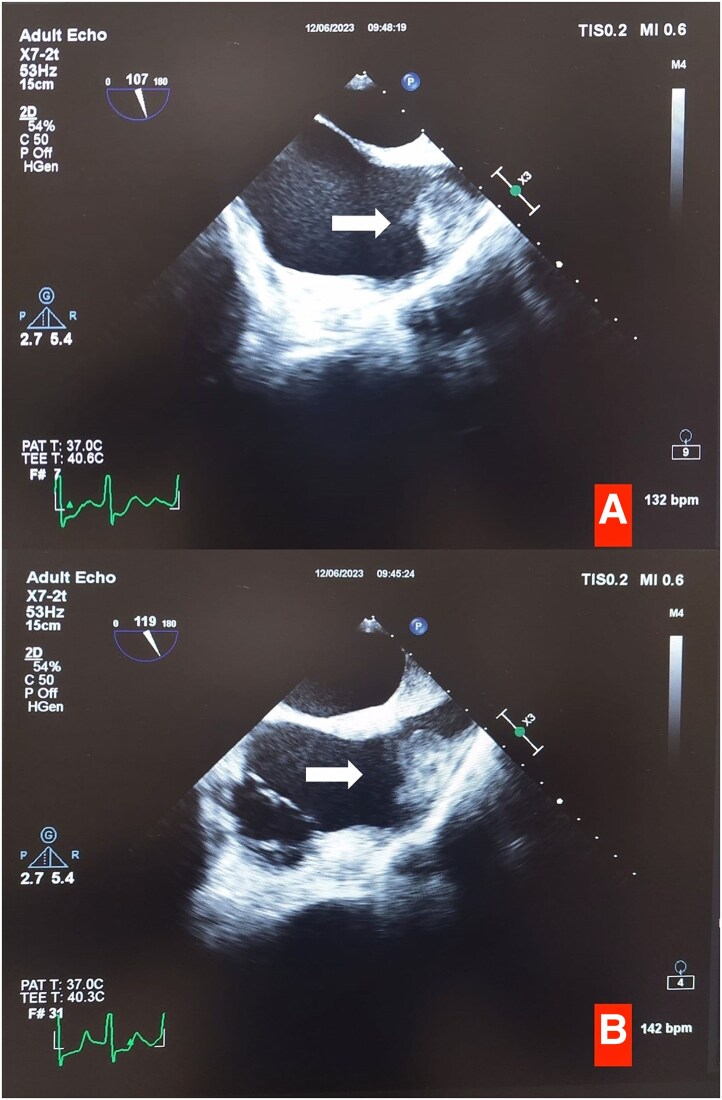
(*A*) Appearance of a mass extending from the right atrium to the right ventricular free wall in transoesophageal echocardiography (white arrows). (*B*) Mass extending from the right atrium to the superior vena cava in bicaval view in transoesophageal echocardiography (white arrows).
